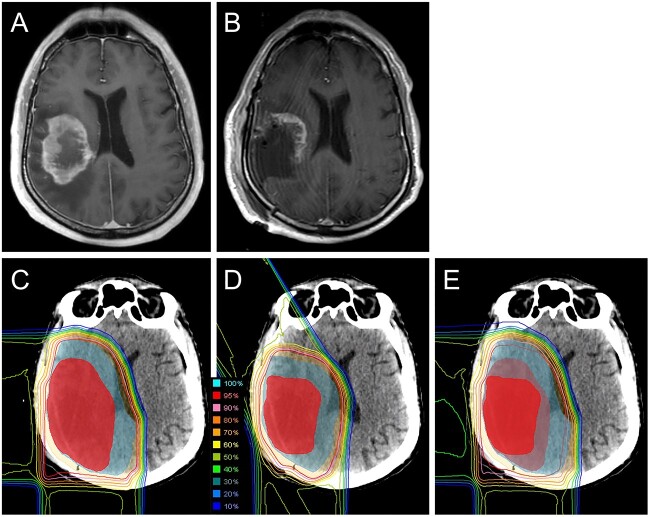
Magnetic resonance images and dose distribution for a patient with glioblastoma of the right frontal lobe. Postcontrast T1-weighted magnetic resonance imaging (MRI) before (A) and after surgery (B). Dose distributions of initial phase with 50 Gy (RBE) in 25 fractions (C), boost with 10 Gy (RBE) in five fractions (D) and the sum of the two with 60 Gy (RBE) in 30 fractions (E).
